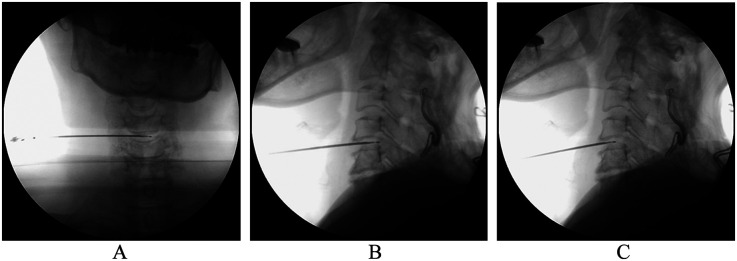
x-ray of cannula position. (A) Anterior–posterior and (B) lateral view of cannulation at initial surgical site. (C) The second site of laser decompression is located in the middle of intervertebral disc, confirmed by the lateral view of fluoroscopy.
